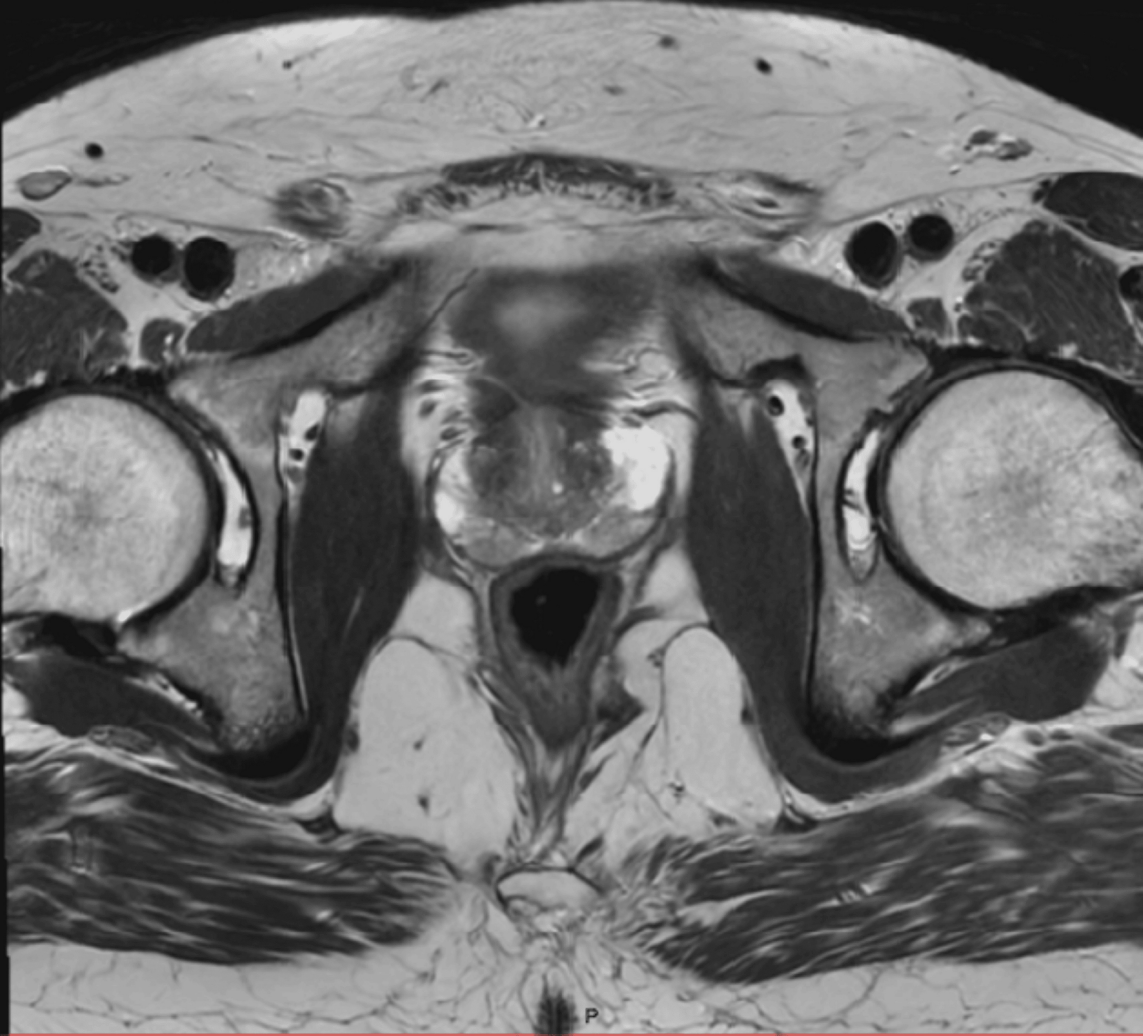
Characteristic appearance of the “organized chaos” in BPH seen on T2WI BPH, benign prostatic hyperplasia; T2WI, T2-weighted imaging
